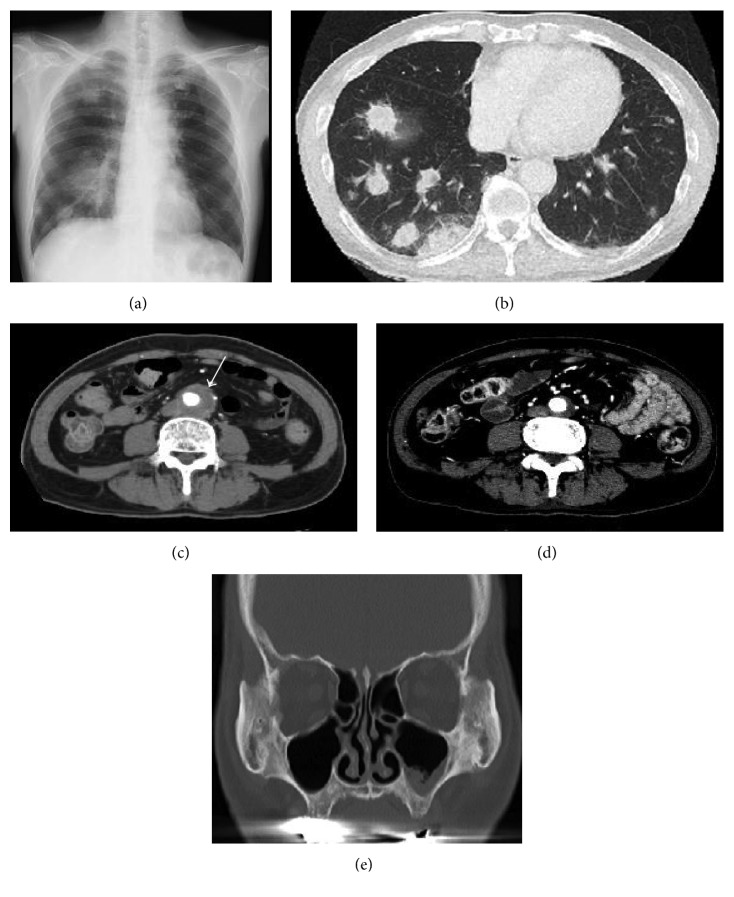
Chest radiograph and computed tomography (CT) of our patient with granulomatosis with polyangiitis. (a) Chest radiography demonstrating lung nodules. (b) CT of the chest showing lung nodules bilaterally. (c) CT of the abdomen showing a localized abdominal aortic aneurysm with a periaortic soft tissue mass. (d) CT of the abdomen after treatment. (e) CT of the left maxillary sinus.
